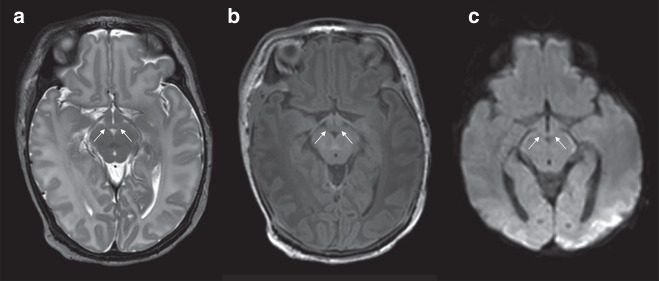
Infant with normal mammillary bodies. Normal mammillary body signal and no swelling on T2 (a), T1 (b) and DWI (c) in a male infant, 41 weeks GA, with perinatal asphyxia and treated with therapeutic hypothermia.
